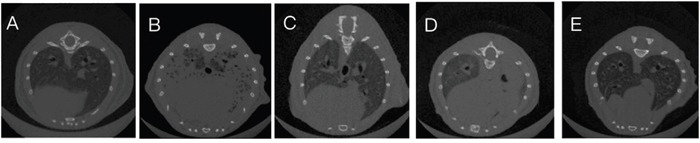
CT scans of lung tissues at 48 h after LPS challenge (A) Control, (B) LPS+PBS, (C) LPS+hUC-MSCs, (D) LPS+FTY720, (E) LPS+ hUC-MSCs+ FTY720.
